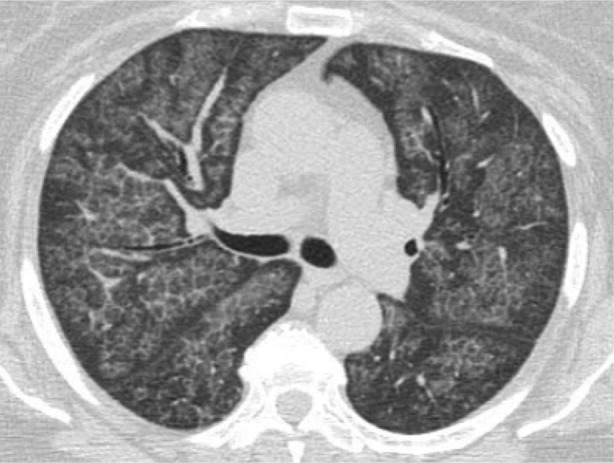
Axial chest high‐resolution computed tomography (HRCT) showing bilateral widespread ground‐glass opacification with superimposed septal thickening referred to as “crazy‐paving” appearance.
